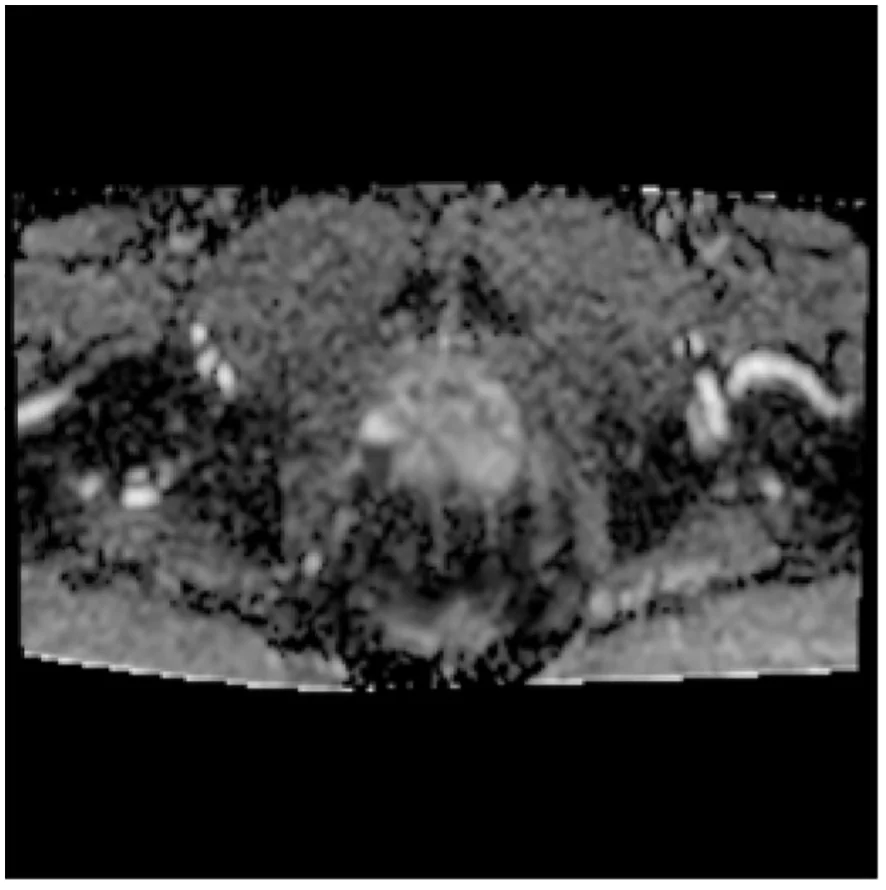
Show MRI image of a patient on ADC. Image preprocessing and analysis masks.
